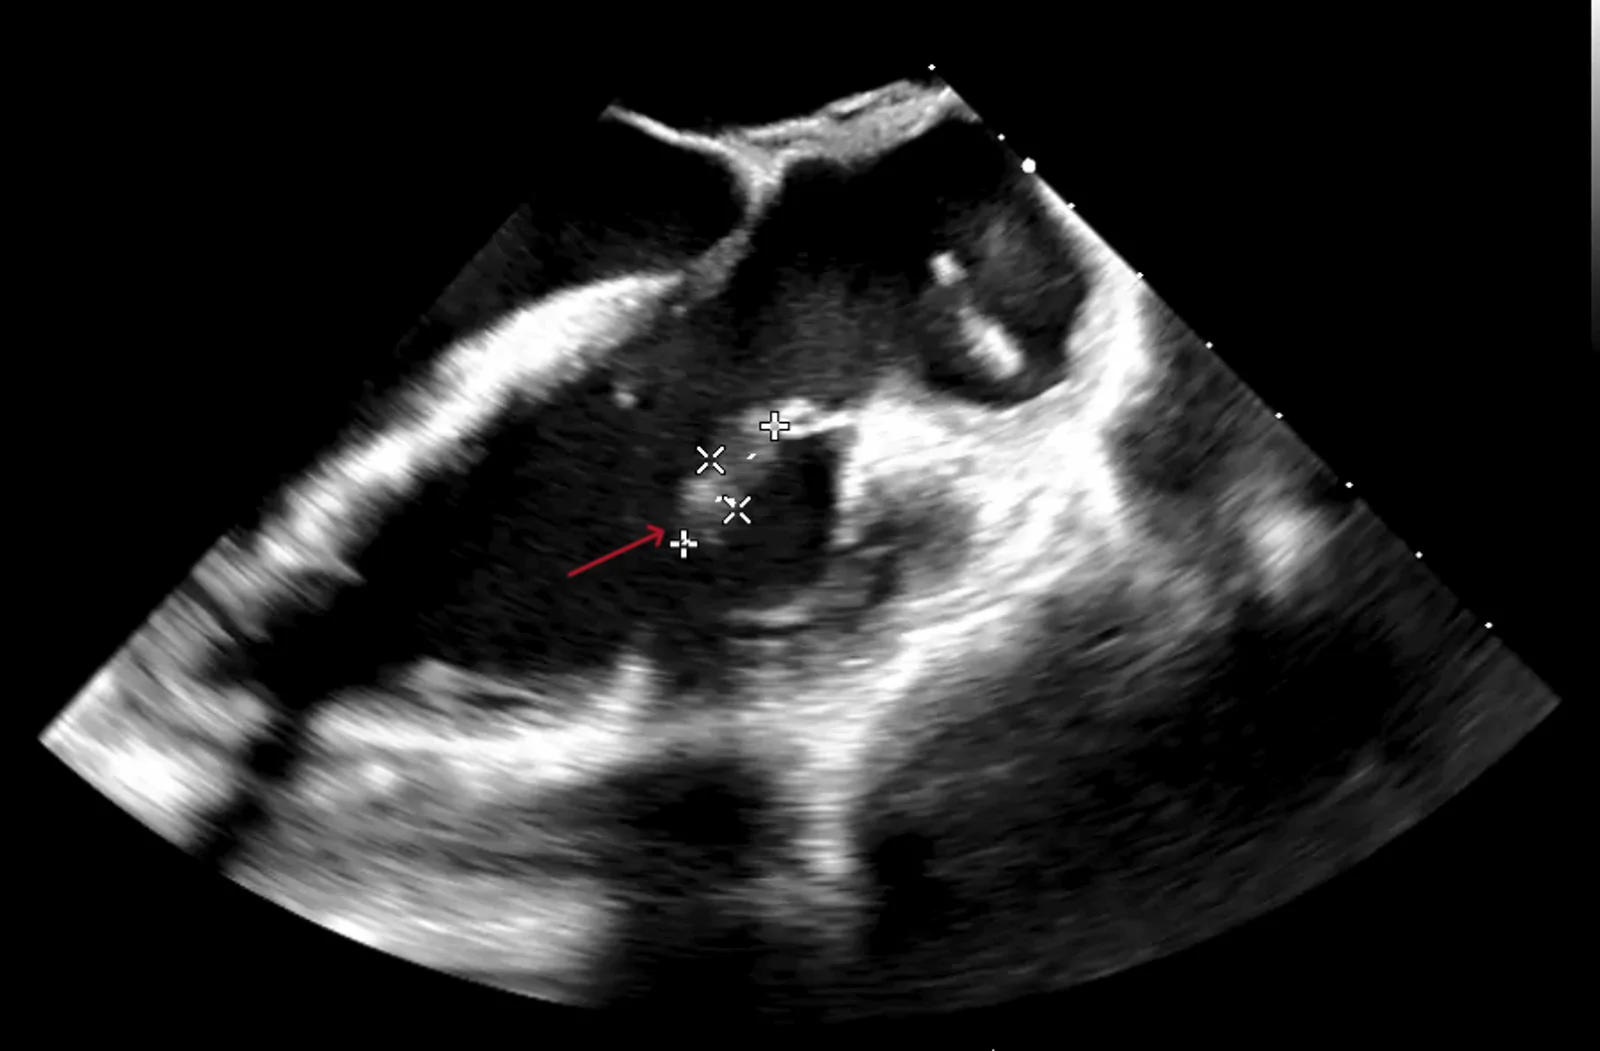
Transoesophageal echocardiogram (TOE) demonstrating a mobile echogenic mass on the posterior mitral valve leaflet in keeping with a vegetation measuring 0.8 cm x 0.28 cm (red arrow) (apical 2-chamber view).
